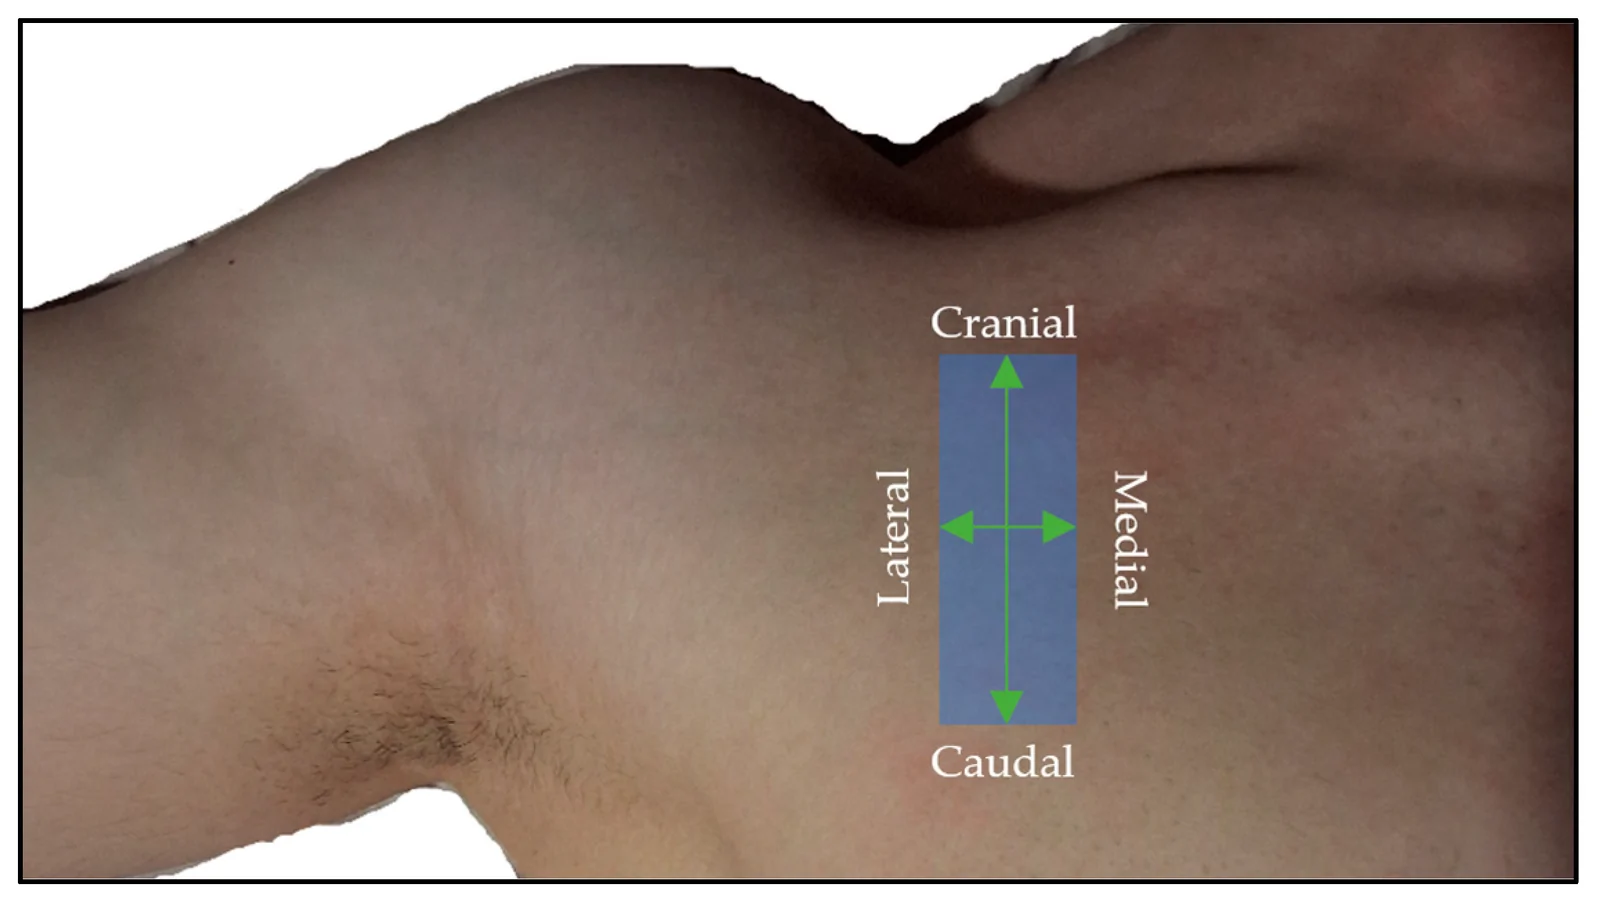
Transducer position for infraclavicular ultrasonographic examination. The linear transducer was positioned in the mid-infraclavicular region, approximately beneath the middle portion of the clavicle, in a parasagittal craniocaudal orientation. The blue rectangle indicates the approximate transducer position, and the arrows indicate the cranial, caudal, medial, and lateral directions.
